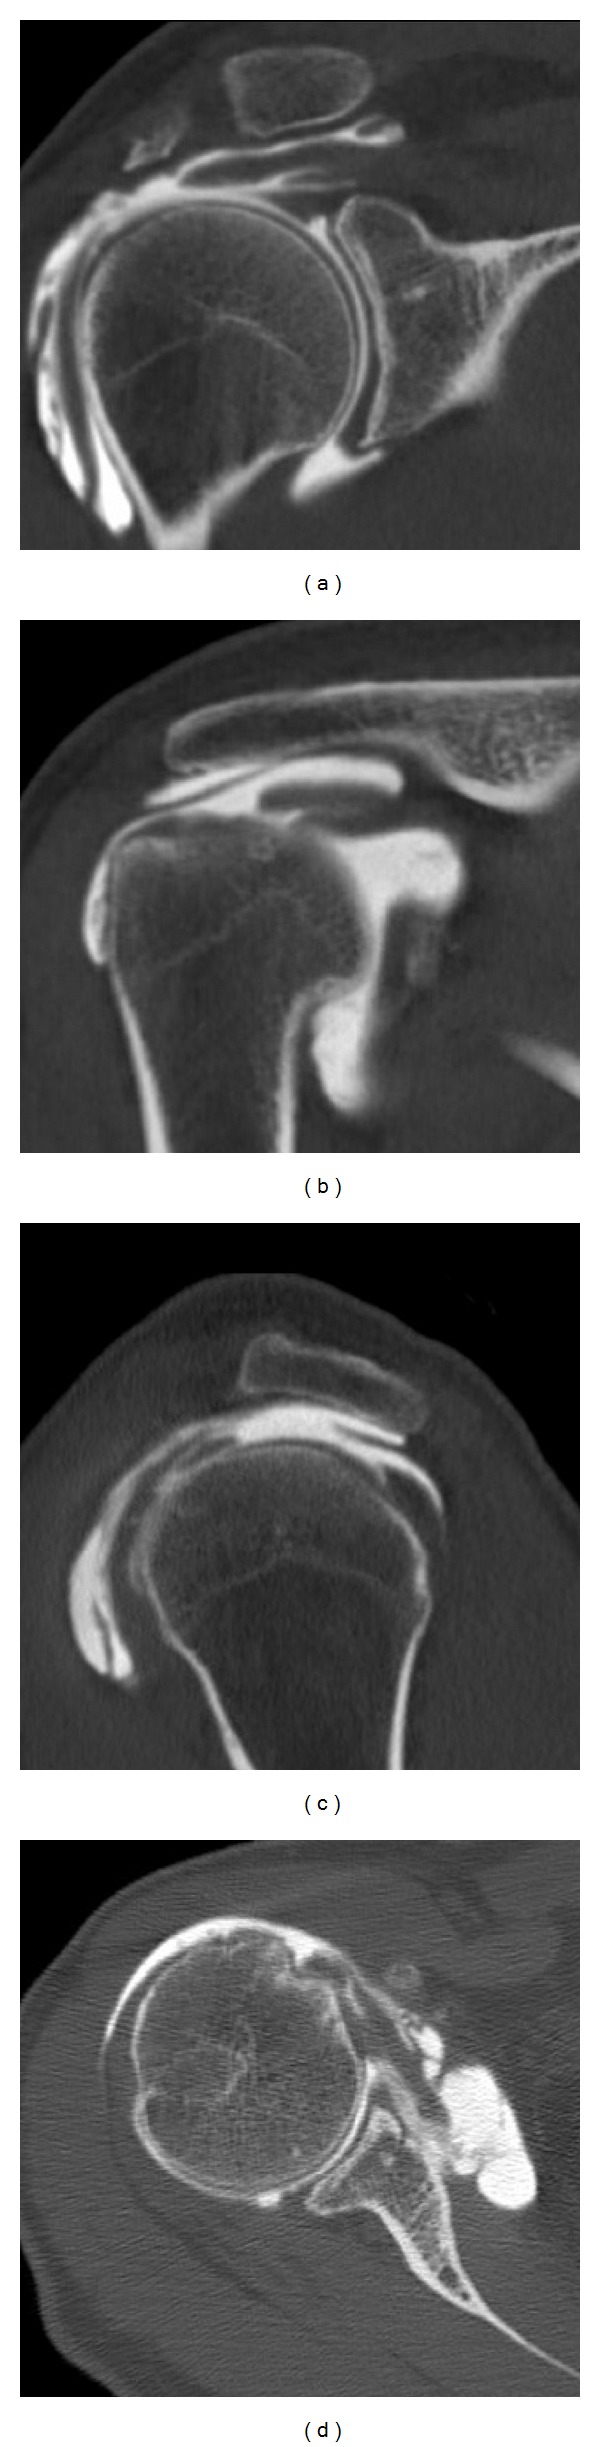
Patient 2 had the following CT arthrogram at time of presentation. It confirmed the exam findings of torn subscapularis, supraspinatus, and infraspinatus. Biceps tendon was diseased.
